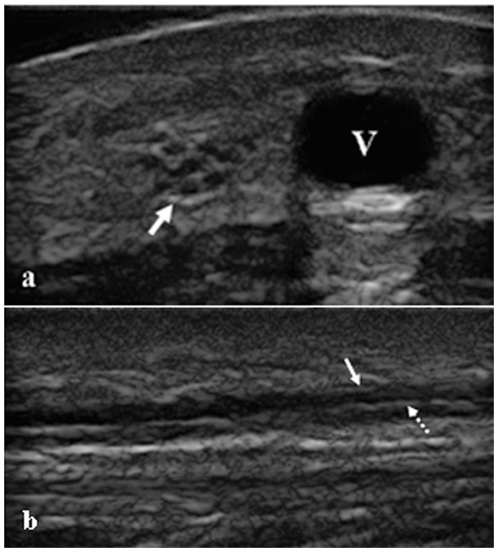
Transverse and longitudinal sonograms of the SN. a) Transverse sonogram of the SN. A transverse sonogram showing the SN (arrows) near the lesser saphenous vein (V) SN appeared as an ovoid hypoechoic reticular structure with a hyperechoic rim. b) Longitudinal sonogram of the SN. A Longitudinal sonogram of the SN showing the perineurium (dashed arrows) and epineurium (solid arrow).
